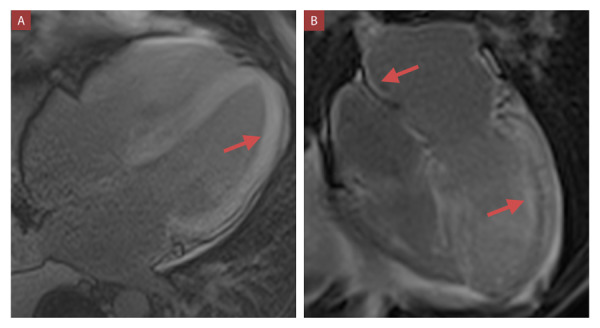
Resonancia magnética cardíaca en vista apical 4 cámaras que muestra captación con realce tardío de gadolinio (flecha roja): A) Patrón transmural ventricular. (Caso 5) B) Patrón subendocárdico difuso biventricular, del septum interauricular y de las paredes auriculares (flechas rojas). Además se observa la anulación del pool sanguíneo. (Caso 6)
